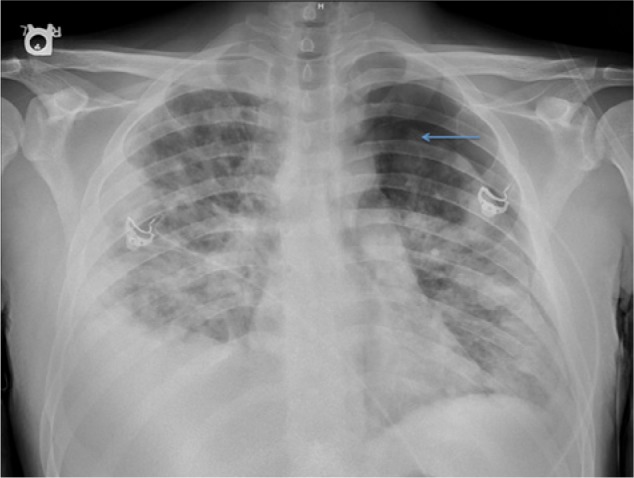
Chest x-ray showing bilateral infiltrates, left pneumothorax, and right pleural effusion. Pneumothorax (marked as a blue arrow) in the left lung.
